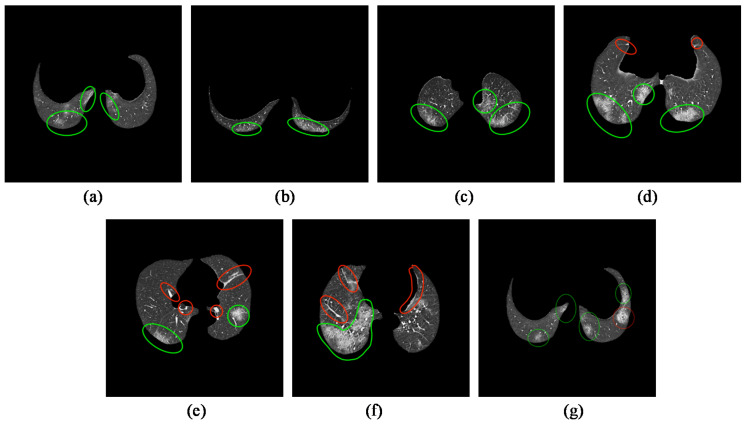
(a–g) Samples of synthetic COVID-19 CT images examined by an experienced radiologist. The green annotations indicate findings of GGO, whereas red annotations indicate irrelevant features of GGO.
